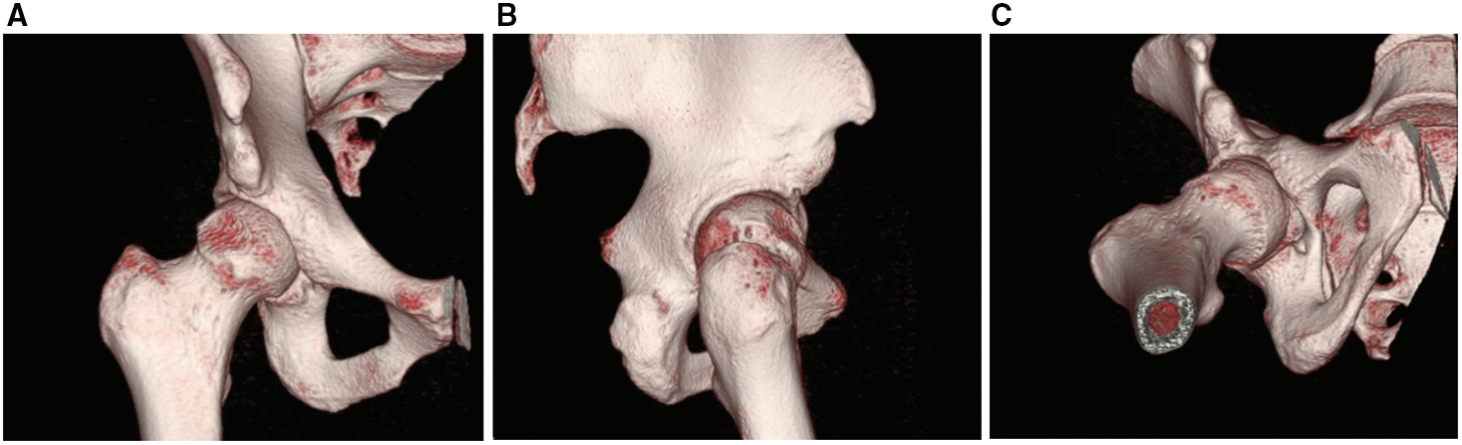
Coronal (A), sagittal (B) and axial (C) view of 3-D CT hip demonstrating post-surgical changes (overly aggressive anterior resection) and inadequately addressed residual cam where the deformity is persistent laterally and distally. Additionally, this patient demonstrates borderline acetabular dysplasia. The 3-D CT can provide invaluable information for preoperative planning.
